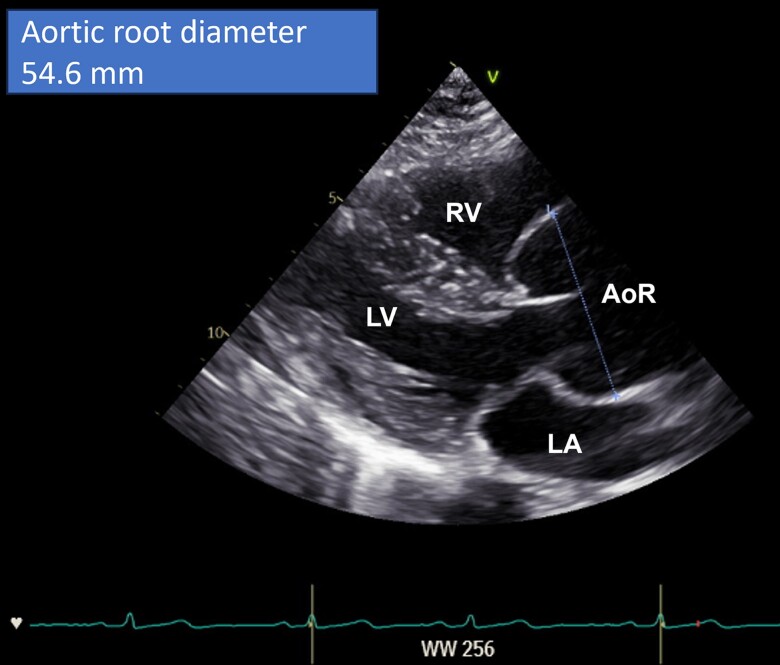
Parasternal long axis view showing the enlarged aortic root (AoR) with a maximum diameter of 55 mm. AoR, aortic root; LA, left atrium; LV, left ventricle; RV, right ventricle.
